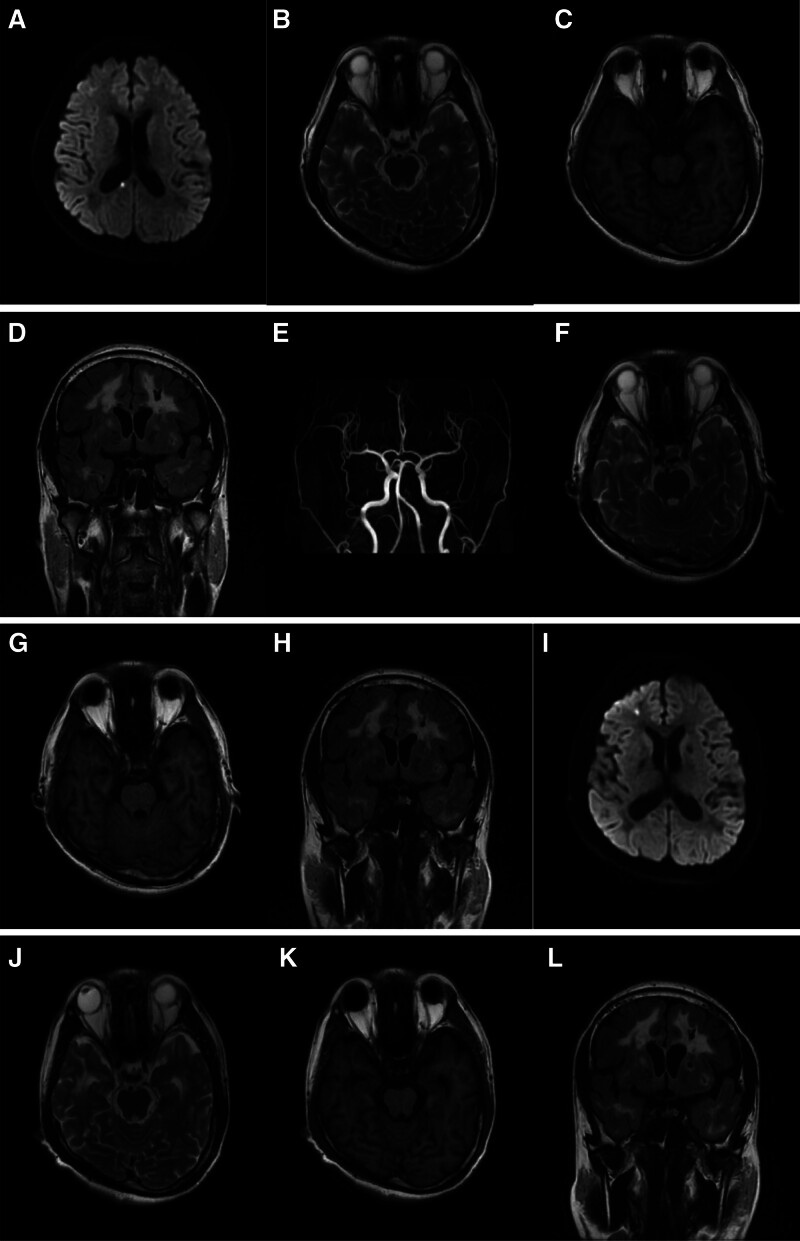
(A) The DWI sequence identified a hyperintense signal in the right corpus callosum, indicative of a potential cerebra acutel ischemic infarction. (B–D) T2-weighted and FLAIR sequences exhibited bilateral, symmetric high-intensity signals around the lateral ventricles and in the deep white matter, along with multiple lacunar strokes involving the external capsule and the temporal pole, with the affected areas presenting slightly hypointense on T1-weighted images. (E) MRA showed no evidence of abnormal changes in the cranial arteries. (F–H) T2-weighted, T1-weighted, and FLAIR sequences displayed an increase in diffuse white matter rarefaction and lacunar infarcts as compared to earlier images (B–D). (I) DWI sequence detected a hyperintense signal in the right frontal lobe, suggestive of an acute cerebral infarction. (J–L) T2-weighted, T1-weighted, and FLAIR sequences revealed a progression in diffuse white matter rarefaction and the number of lacunar infarcts compared to images (F–H). DWI = diffusion weighted imaging, FLAIR = fluid-attenuated inversion recovery, MRA = magnetic resonance angiography.
